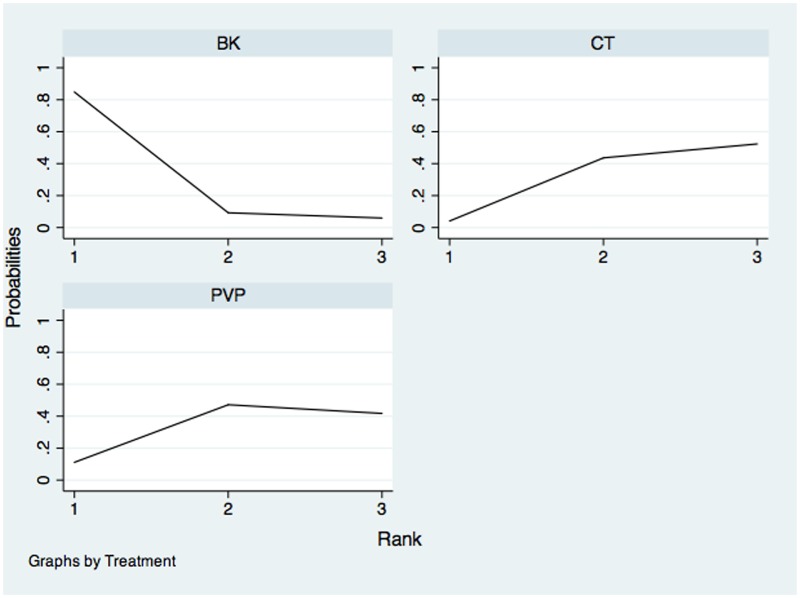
Ranking of treatment strategies based on probability of their effects on outcome of all-cause discontinuation. PVP: Percutaneous vertebroplasty; BK: Balloon kyphoplasty; CT: Conservative treatment.
